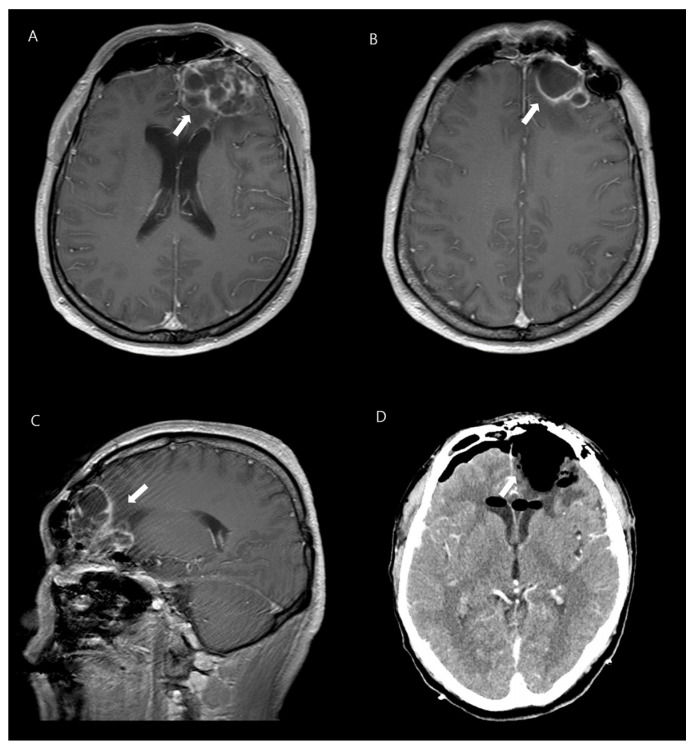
A 42-year-old man with *K. pneumoniae* brain abscesses. Axial contrast-enhanced T1-weighted images (A,B) and sagittal image reveal thin-walled rim-enhancing abscesses in the left frontal lobe (C). Enhanced brain CT shows disappeared lesions after the neurosurgery (D).
